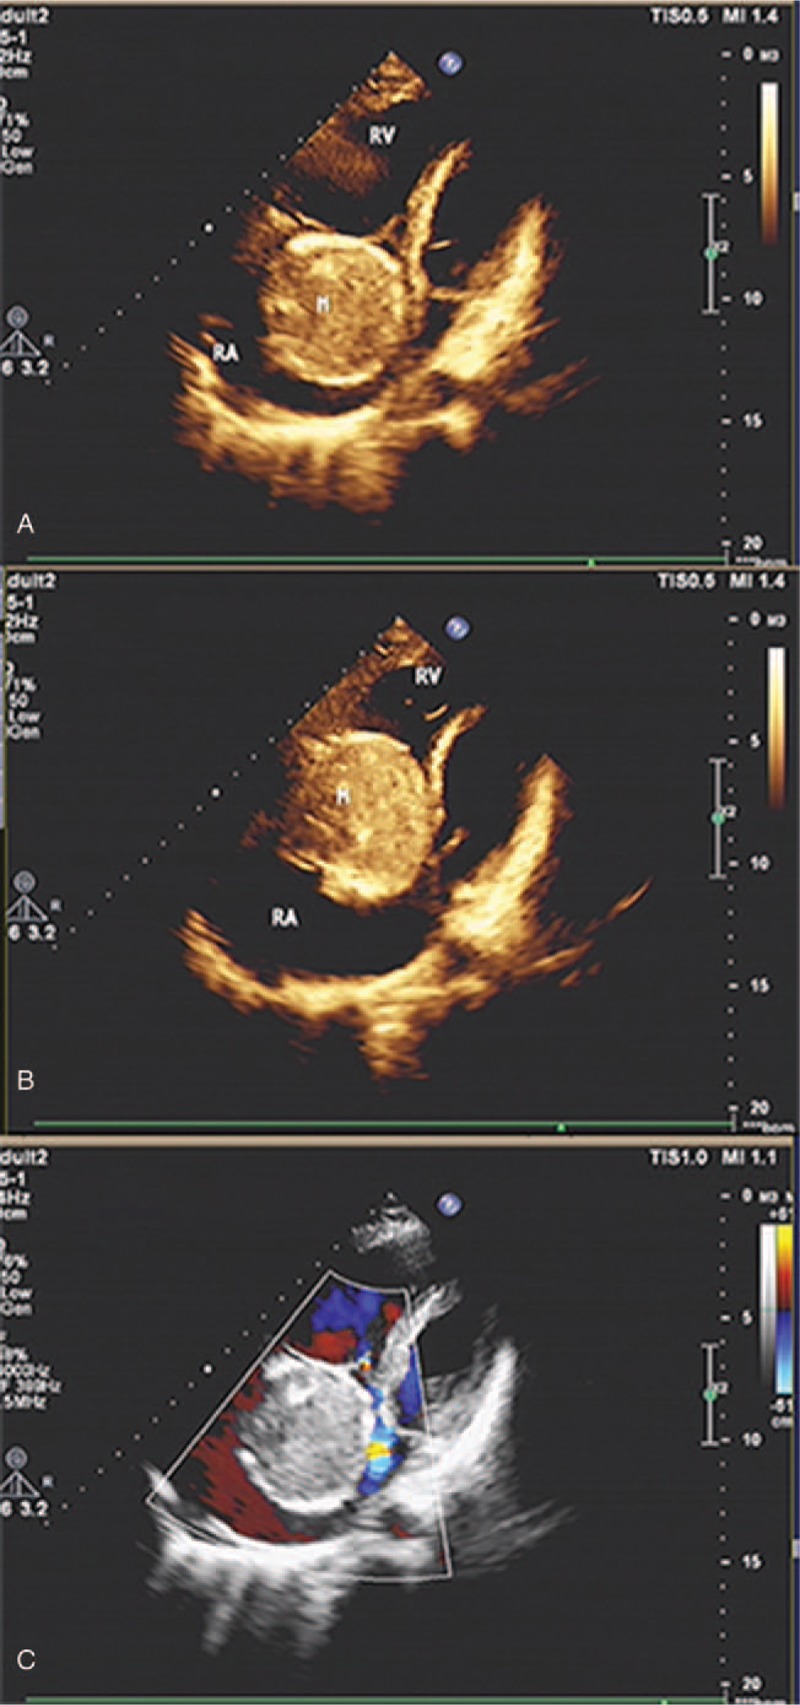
Transthoracic echocardiography demonstrating a huge, regular mass (72 × 58 mm) located at the right atrium in the systole (A) and prolapse to the right ventricle in diastole (B). The tumor causes in mild stenosis and moderate regurgitation of the tricuspid valve (C). M = mass, RA = right atrium, RV = right ventricle.
